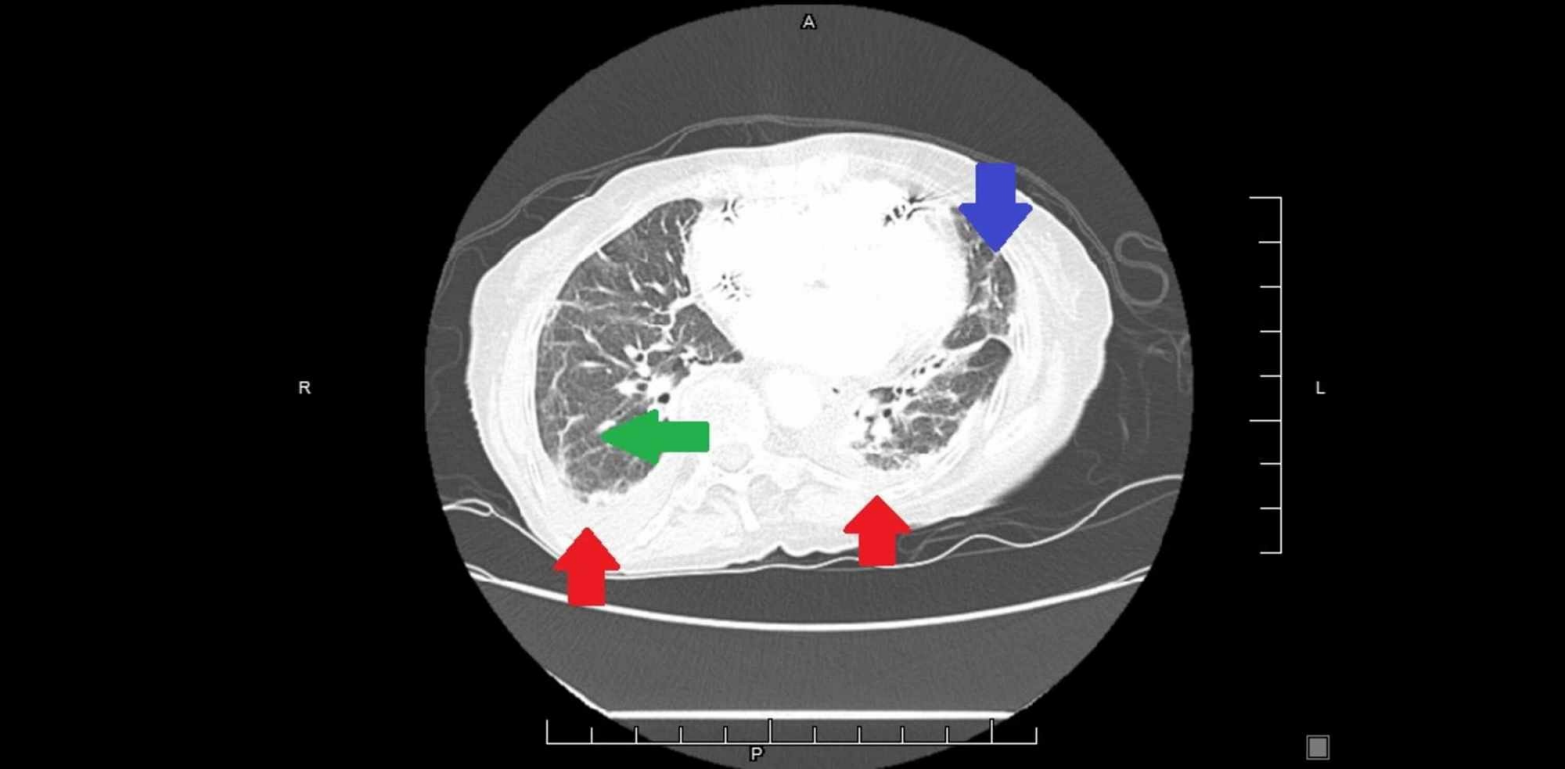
CT angiography of the chest Red arrow - pleural effusion, green arrow - opacity, blue arrow - honeycombing
